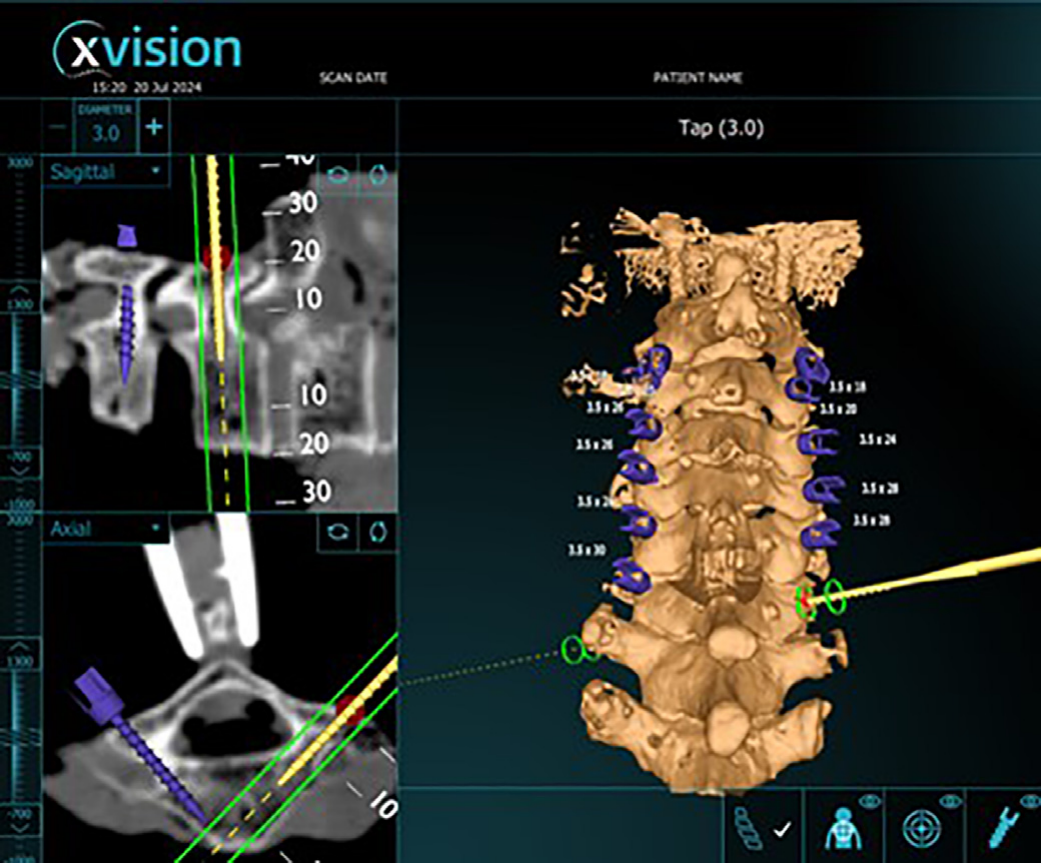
Screen shot showing tap on axial CT scan, sagittal CT scan, and 3D model.
